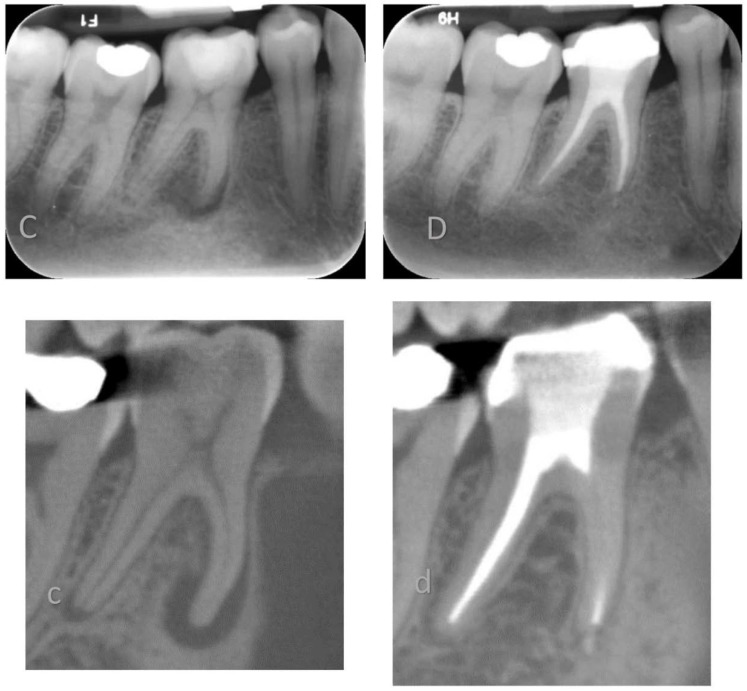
Lower right mandibular first molar root filled using a single cone technique and BioRoot^TM^ RCS. Preoperative PA X-ray (C) and CBCT (c) and recall PA X-ray (D) and CBCT (d) at 12 months.
